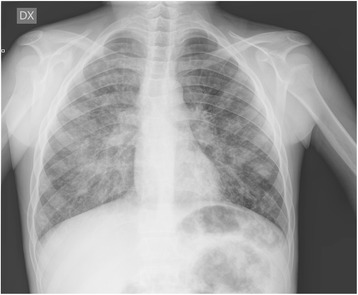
Chest X-ray: multiple bilateral opacities and reticular pattern in both thoracic fields
